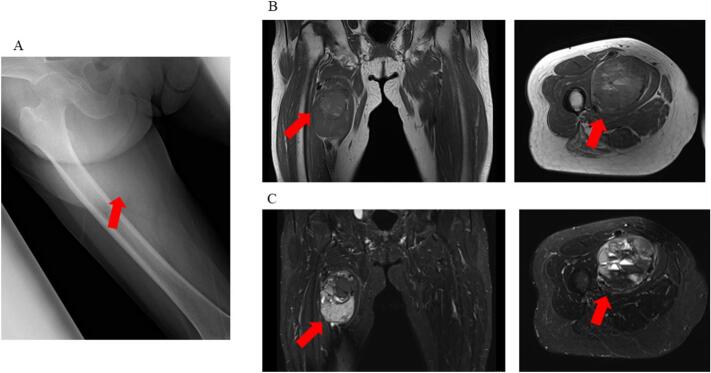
X-ray and preoperative magnetic resonance imaging of ependymoma in the proximal medial thigh. (A) A swollen soft shadow was seen on the medial side of the right thigh. (B) T1-weighted images show a low signal throughout the interior of the tumor (arrowhead). (C) T2-weighted images show a mixture of low- and high-signal areas inside the tumor, and multiple niveau formations were also observed (arrowhead).
